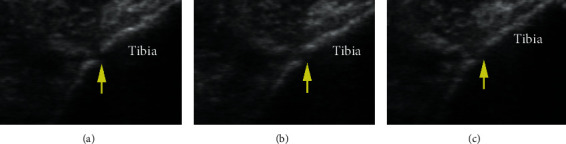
Ultrasound images at the right distal tibia examined, respectively, (a) 2 weeks, (b) 4 weeks, and (c) 6 weeks after the commence of LIPUS therapy. The arrow indicates the defect of the tibia.
